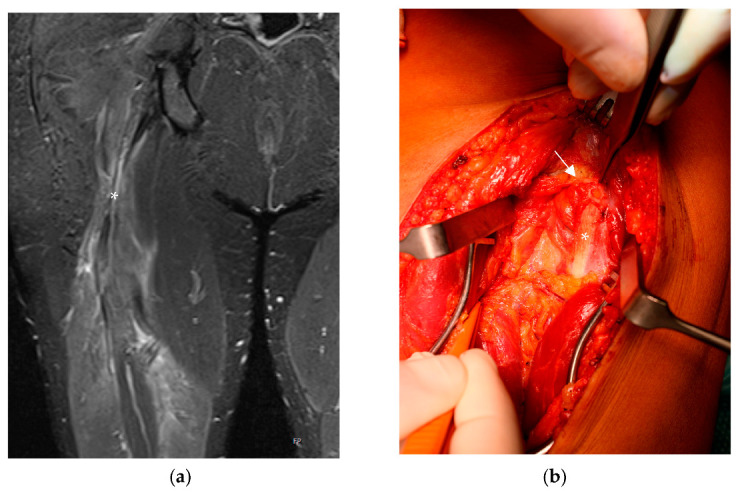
(a) MRI of the right thigh (coronal plane). Hematoma around the sciatic nerve (*) and retraction of the long head of the biceps femoris. (b) Intraoperative picture taken during neurolysis of the sciatic nerve showing the massive scar formation (→) around the entrapped nerve (*).
